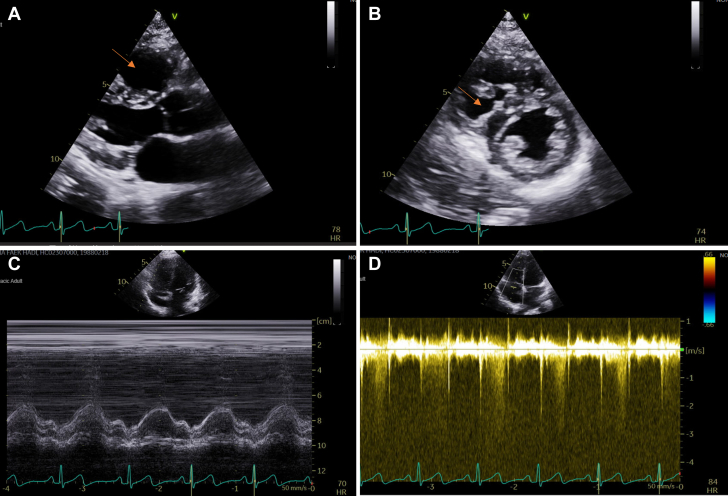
Transthoracic Echocardiographic Images (A, B) A mildly dilated right ventricle (RV) (orange arrow) with mild systolic septal flattening, suggestive of pressure overload. (C) Continuous-wave Doppler signal across the tricuspid valve demonstrating an estimated right ventricular systolic pressure of 53 mm Hg. (D) Assessment of RV function showing a borderline normal tricuspid annular plane systolic excursion of 17 mm. Regional wall motion analysis indicates borderline reduced systolic function at the apex and midsegment of the RV free wall.
